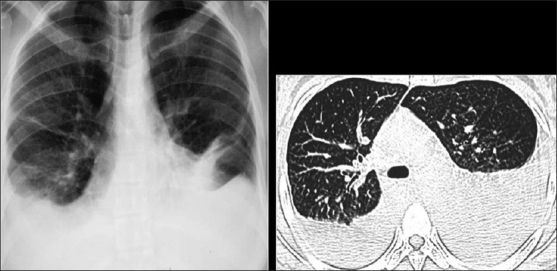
Kaposi sarcoma. Pleural effusions chest X-ray/computed tomography are common. They may be unilateral or bilateral and may be large and characteristically hemorrhagic on aspiration
